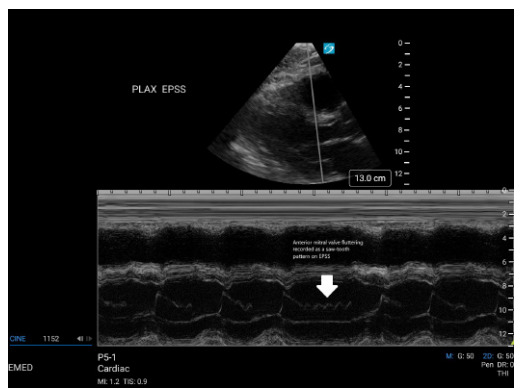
M-mode visualization of anterior mitral valve fluttering in parasternal long axis shows a sawtooth pattern (arrow). *PLAX EPSS*, E-point septal separation measured in the parasternal long-axis view.
